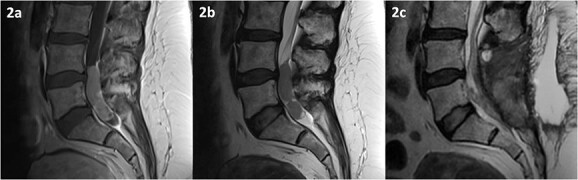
MRI of the spine depicting an intradural lesion between L4-S1. T1-weighted imaging (a) shows heterogeneous enhancement of the lesion upon administration of gadolinium. The lesion is hypointense on T2-weighted imaging (b). Postoperative T2-weighted imaging (c) reveals a small amount of residual tumour.
